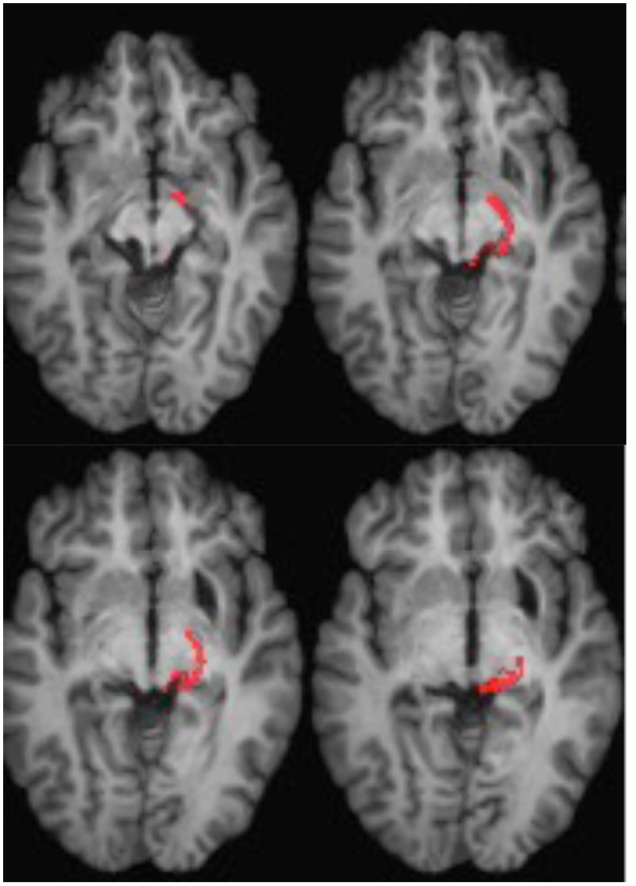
DTI tractography in patient ML showing the retino-tectal tract in the right hemisphere (red) co-registered to T1-weighted MR images in the axial plane from ventral (Top left) to dorsal (Bottom right). Diffusion-weighted echo-planar magnetic resonance images were acquired at 2 mm^3^ resolution with 63 isotropically distributed diffusion-encoding directions (*b* = 1000) and a baseline (*b* = 0). Repetition time = 2 s, and echo time = 35 ms. Tractography was implemented as described in Figure 2.
